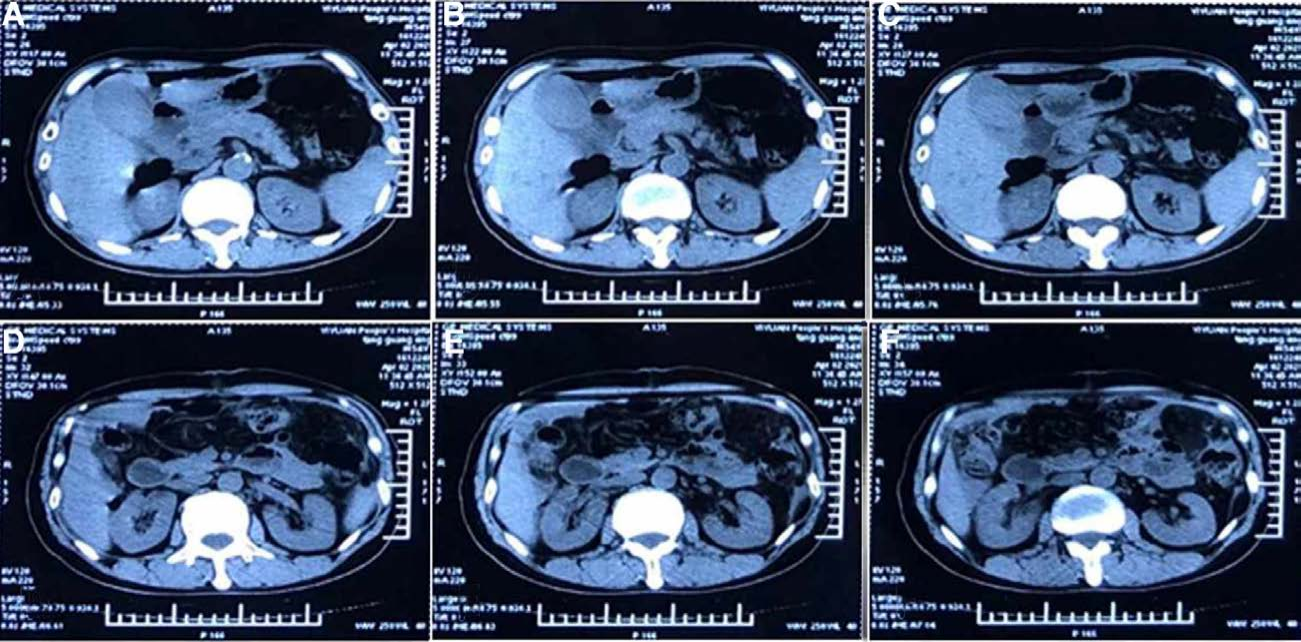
(A–F): Abdominal CT with enhancement: uneven thickening of intestinal wall, consistent with malignant tumor. CT = computed tomography.
